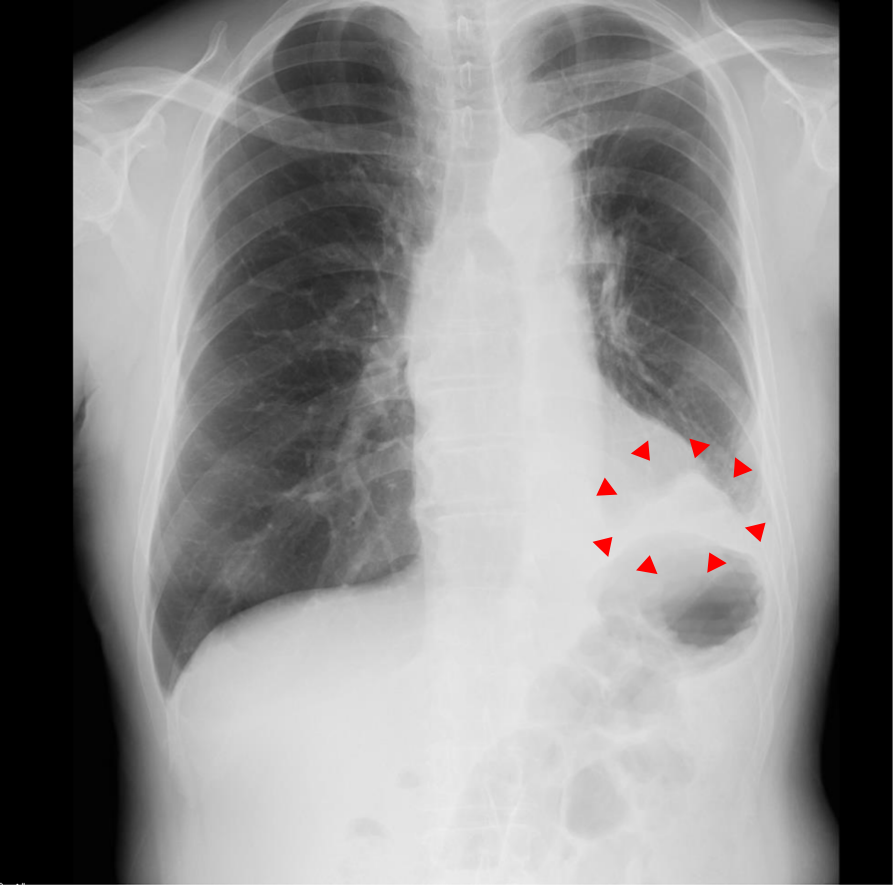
Chest X-ray after two months edoxaban was restarted
